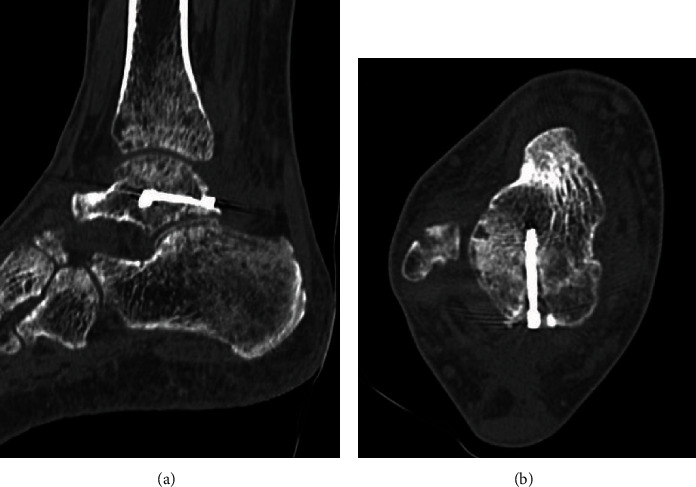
Noncontrast CT in (a) sagittal and (b) axial views at 14 weeks after the operation demonstrated complete bone union.
